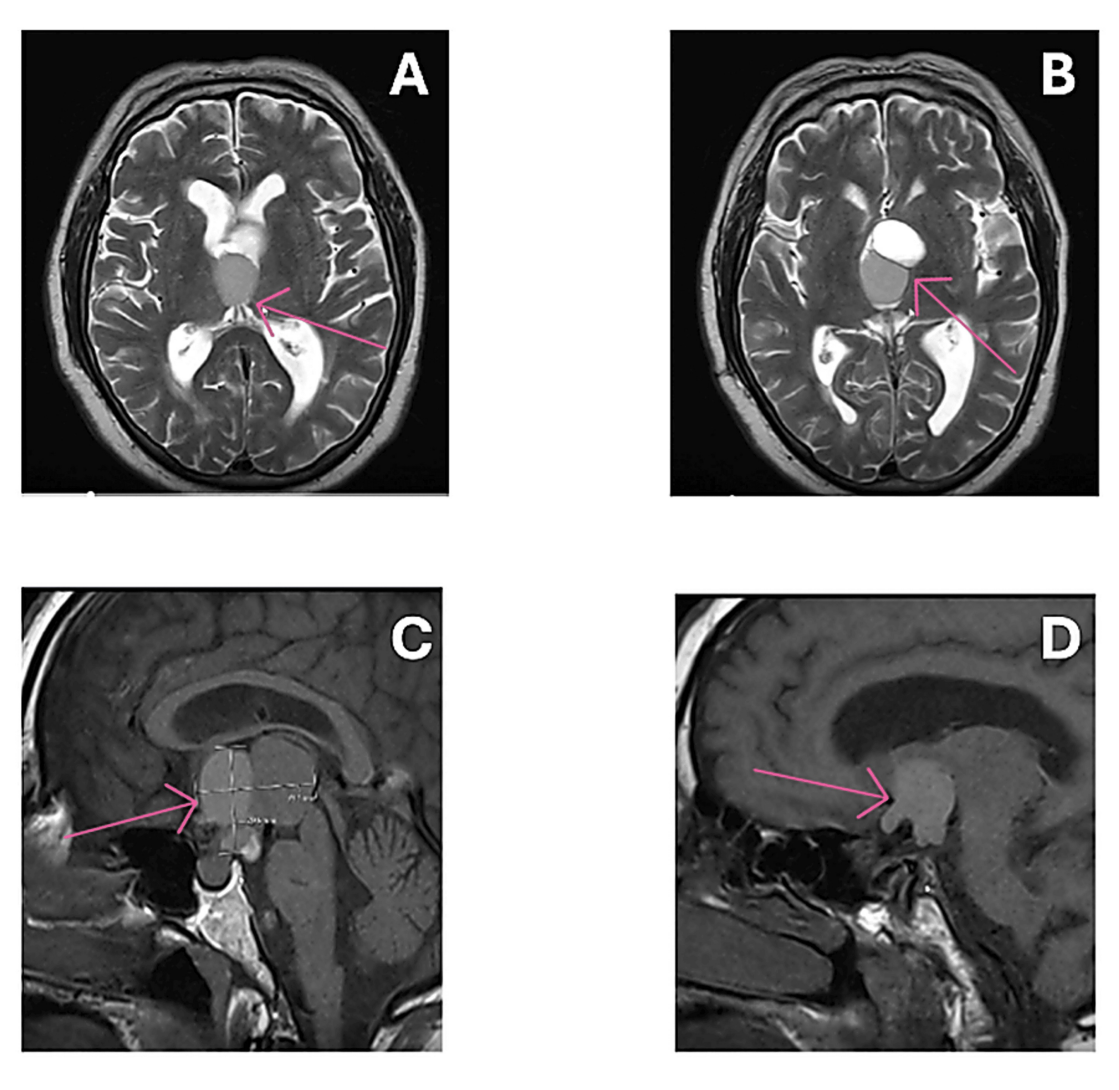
MRI scans prior to the second operation (trans-sphenoidal tumour resection), showing some progression of the disease, with an increase in the size of the craniopharyngioma. A and B: show the presence of the cystic craniopharyngioma in the suprasellar region, depicting the tumour on an axial slice. C and D: further characterise the craniopharyngioma tumour on a sagittal MRI slice. At this point, the patient's symptoms had been progressing slightly, warranting a definitive surgical resection, following the initial transventricular aspiration of the craniopharyngioma cyst a few months before.
